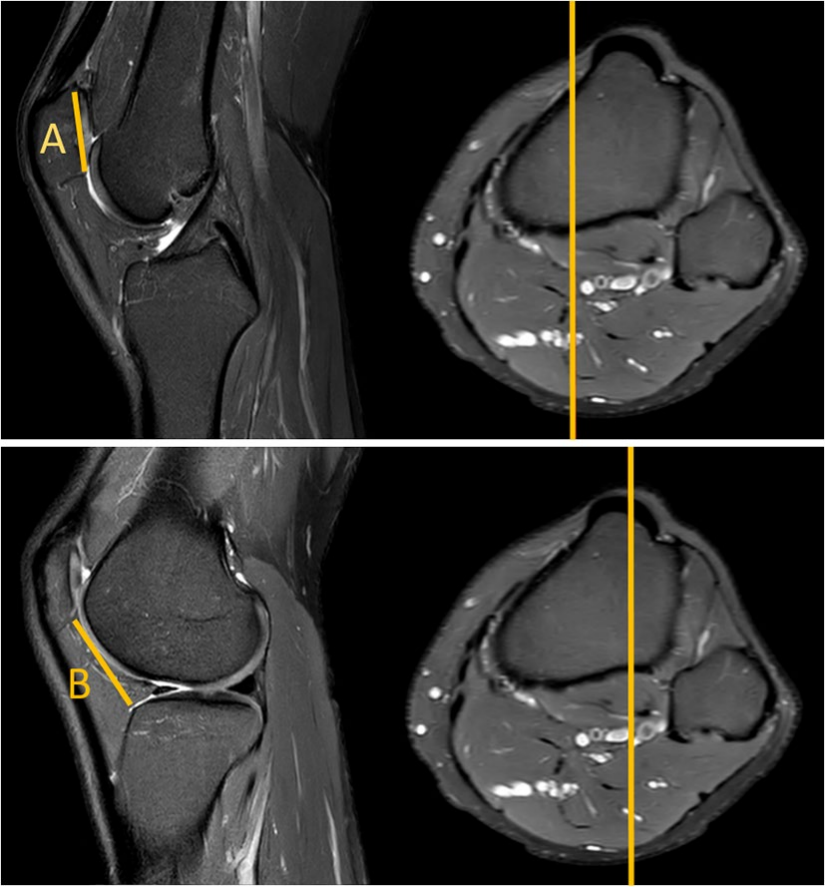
MRI true sagittal view. Caton–Deschamps (CD) index measurement in two slices. A patellar cartilage length (measured in slice with the maximum length of the articular surface of the patella) B distance from Line A to the tibia (measured in slice going through the centre of the tibial tuberosity). CD: B/A
